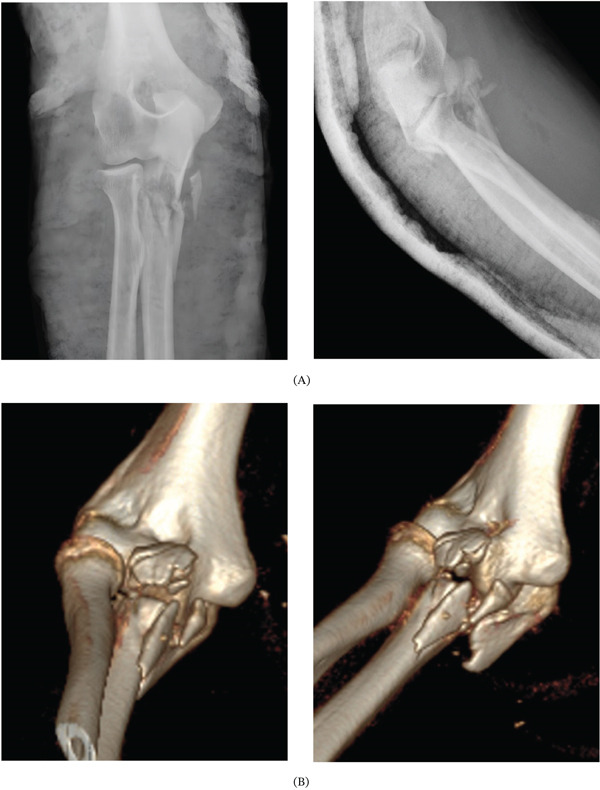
Preoperative radiographic and computed tomography (CT) findings of the right elbow. (A) Anteroposterior and lateral digital radiographs (DR) demonstrating a complex fracture of the proximal ulna involving both the coronoid process and the olecranon, with displacement of the coronoid fragment. (B) Three‐dimensional CT reconstructions providing detailed visualization of the comminuted coronoid process fracture (O′Driscoll Type III) and the associated transolecranon fracture.
